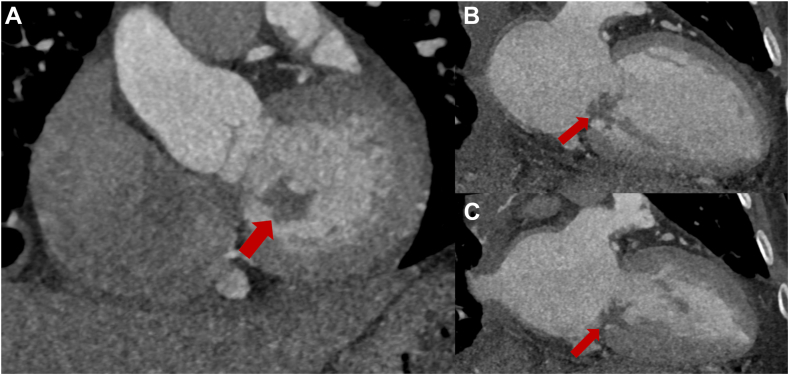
Cardiac CT, multiplanar reconstruction, oblique sagittal (A, basal cardiac short axis), oblique coronal long axis (cardiac 2-chamber view) in diastole (B) and systole (C), demonstrates normal left ventricular size and function and the irregular-shaped, large mass (vegetation) attached to the PMVL.
